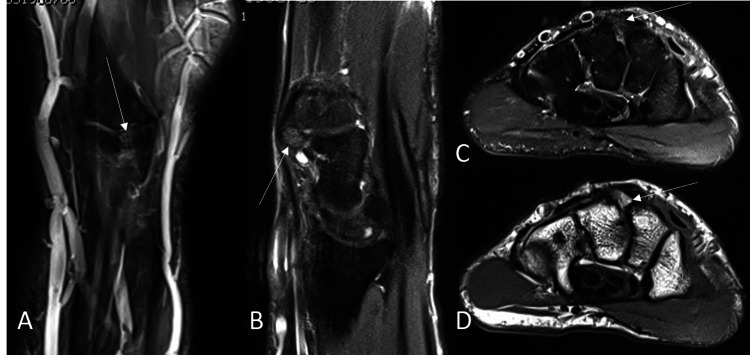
Typical carpal os styloideum. A 39-year-old male with dorsal left wrist pain. Proton-density fat-saturated (PDFS) MRI in coronal (A), sagittal (B), and transverse (C) plane, as well as T1-weighted transverse image (D), demonstrate a small bony fragment at the dorsal aspect of the wrist between the second and third carpometacarpal joint (arrows). Note the high signal intensity of the fragment on PDFS images indicating bone marrow edema of the fragment.
